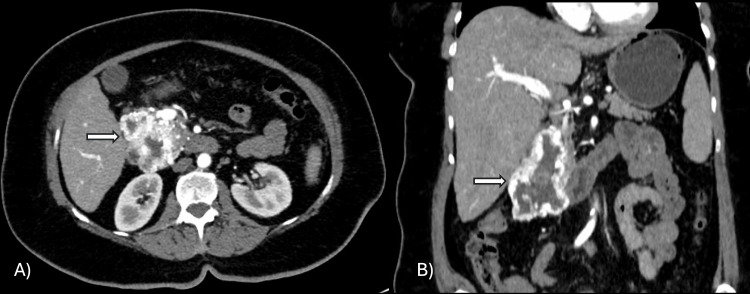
Contrast-enhanced CT scan of the abdomen in the arterial phase Axial (A) and coronal (B) sections showing a solid hypervascular mass in the pancreatic head with irregular margins (arrow), peripheral enhancement, and a large central low-attenuation area compatible with necrosis. CT: computed tomography
